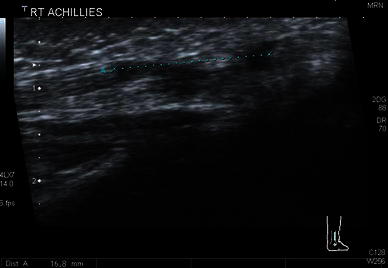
Ultrasound measurement of the tendon gap of the same tendon as Fig. 4 but with the foot in full dorsiflexion. There is a large variation in gap measurement which is greatly affected by the position of the foot
